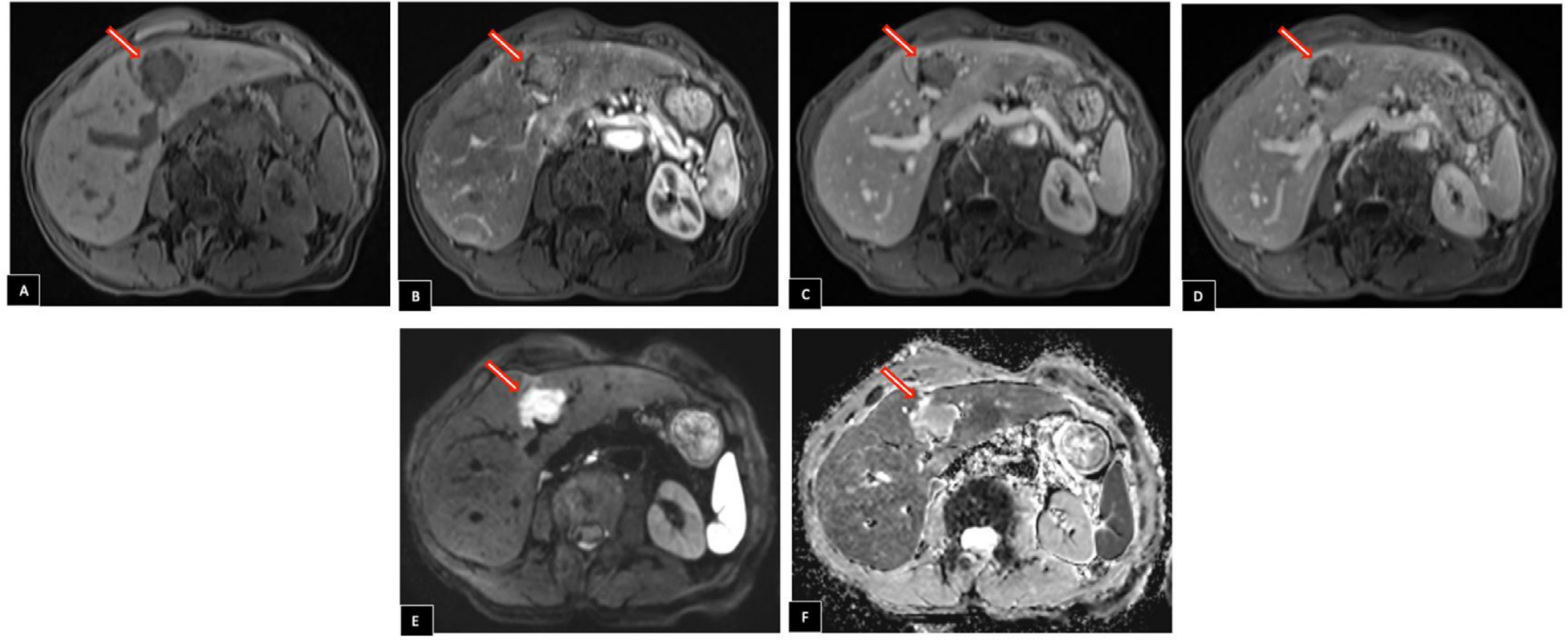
Liver MRI Imaging Findings. (A) Before contrast injection, the mass appears hypointense on T1-weighted imaging. (B) After contrast administration, it demonstrates arterial phase enhancement, which (C, D) persists slightly in the venous phases. (E) Axial diffusion-weighted imaging shows high signal intensity in the mass with (F) corresponding low signal intensity on the apparent diffusion coefficient (ADC) map. The mass and its characteristics are indicated by the arrows.
